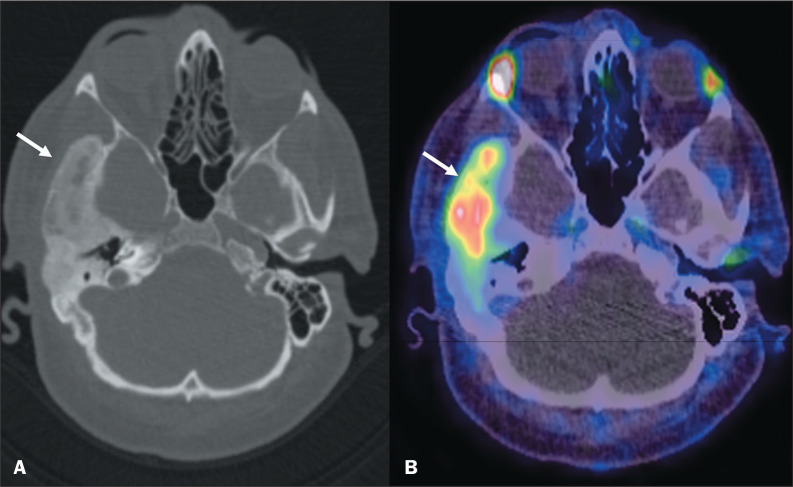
Preoperative ^68^Ga-PSMA PET/CT of a 58-year-old male
